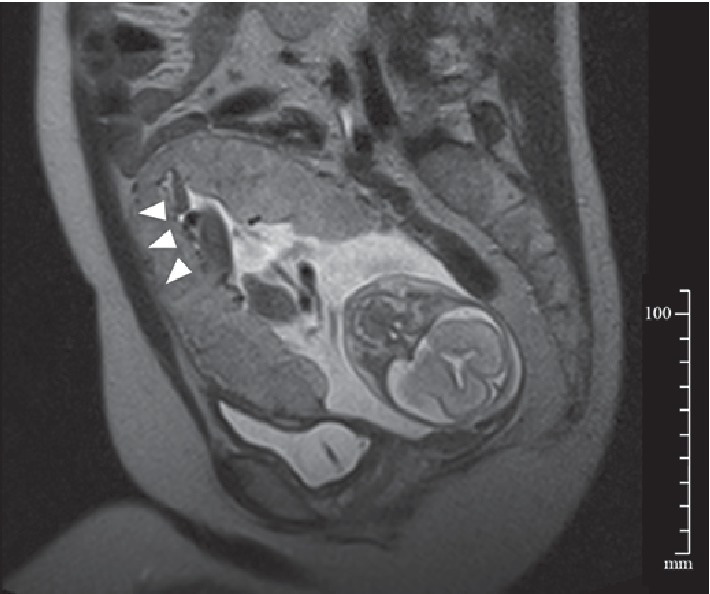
Sagittal T2-weighted magnetic resonance image of the uterus at 22^+4^ weeks of gestation. The myometrium is extremely thin throughout the uterus with partial bulging of the anterior uterine wall (arrowheads).
